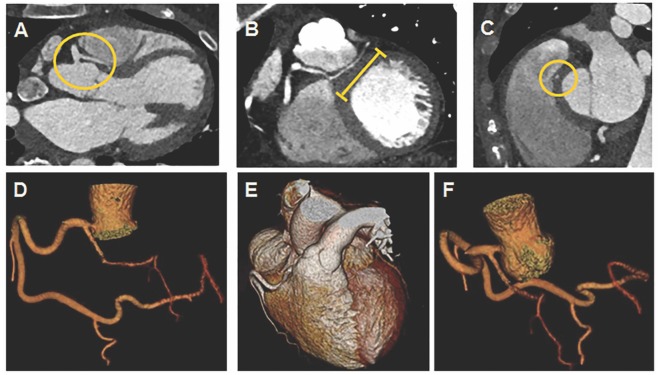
Coronary computed tomography angiogram (CTA) with 3D reconstruction. (A) Yellow circle denotes LAD anomalously arising from proximal RCA without a slit like orifice and coursing between aortic root and RVOT. (B) Yellow line denotes LAD with an intra-myocardial segment. (C) Yellow circle denotes LAD with an intra-myocardial segment in cross section. (D,E) RCA coursing into left atrioventricular groove to supply circumflex artery. (F) LAD arising anomalously from proximal RCA.
